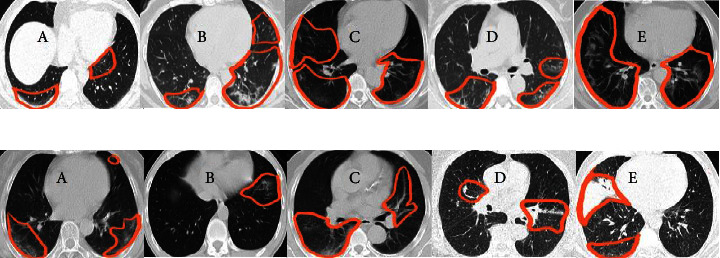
Examples of misclassified slices by the COVID-19 models. (a) COVID-19 CT slices were classified as non-COVID-19. Marked areas represent: in (A) GGO (B) GGO and consolidation (C–E) GGO. (b) Non-COVID slices were classified as COVID-19. Marked areas represent: in (A, B) GGO (C) GGO and vascular enlargement (D, E) FB.
